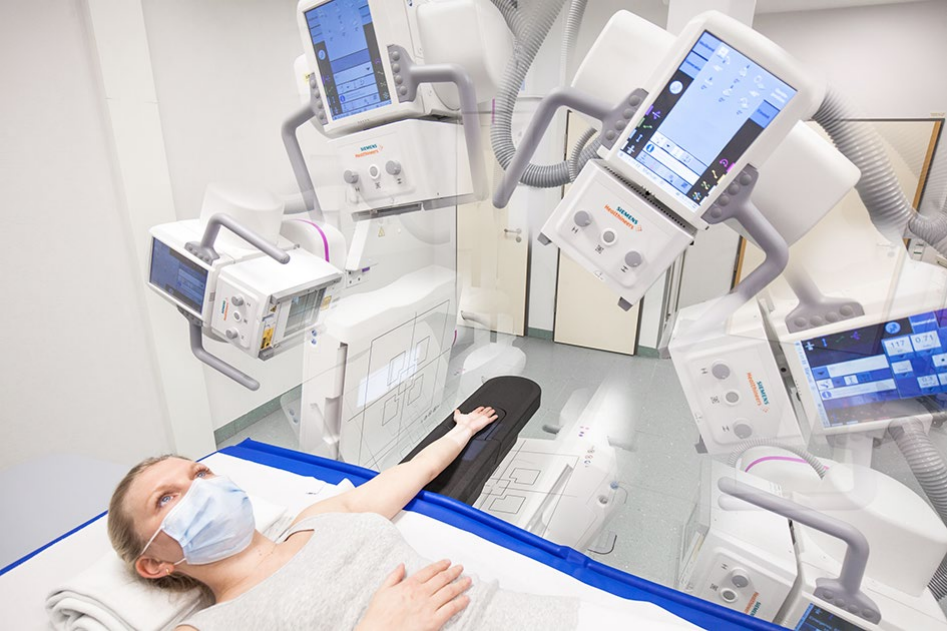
Rendering of staff member demonstrating the scan position for 3D cone-beam CT imaging of upper extremity joints using the tableside trajectory of the twin robotic x-ray system.
